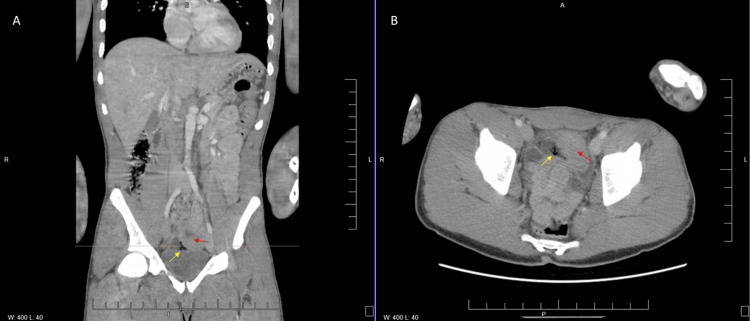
CT abdomen and pelvis with contrast from February 22, 2023, demonstrating air bubbles and bowel thickening A) CT abdomen and pelvis coronal view. The dotted orange line indicates the corresponding level in Panel B. Yellow arrows indicate a free-air bubble. Red arrows indicate bowel thickening. B) CT abdomen and pelvis axial view.
